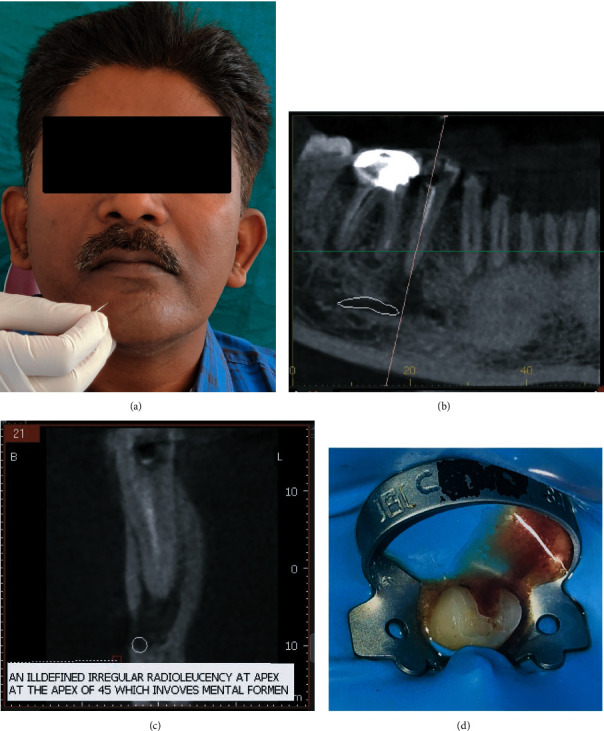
(a) Paraesthesia testing, (b, c) preoperative CBCT, and (d) access cavity under rubber dam isolation.
